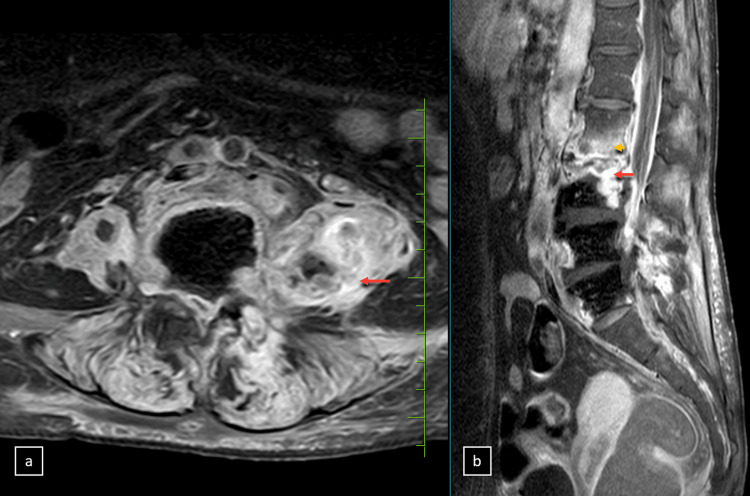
Magnetic resonance imaging (MRI). (a) Axial (b) and sagittal sections of post-contrast images show peripheral enhancement around fluid collections, enhancement of paravertebral soft tissues (red arrows), and enhancement of vertebral endplates (orange arrowheads).
